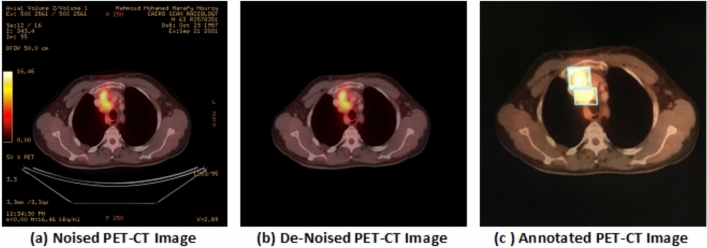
The noise removal step of PET-CT images.
